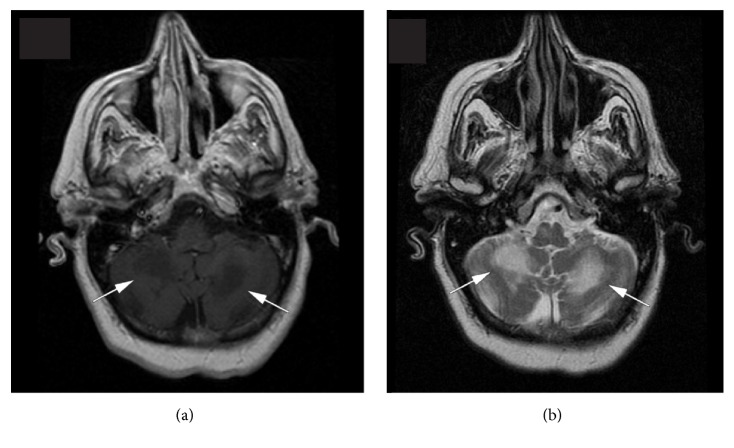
While the brain parenchyma was normal, the MRI shows asymmetric hypointense lesions in both cerebellar hemispheres on T1-weighted images (Slide (a)) and hyperintense lesions on T2-weighted images (Slide (b)).
